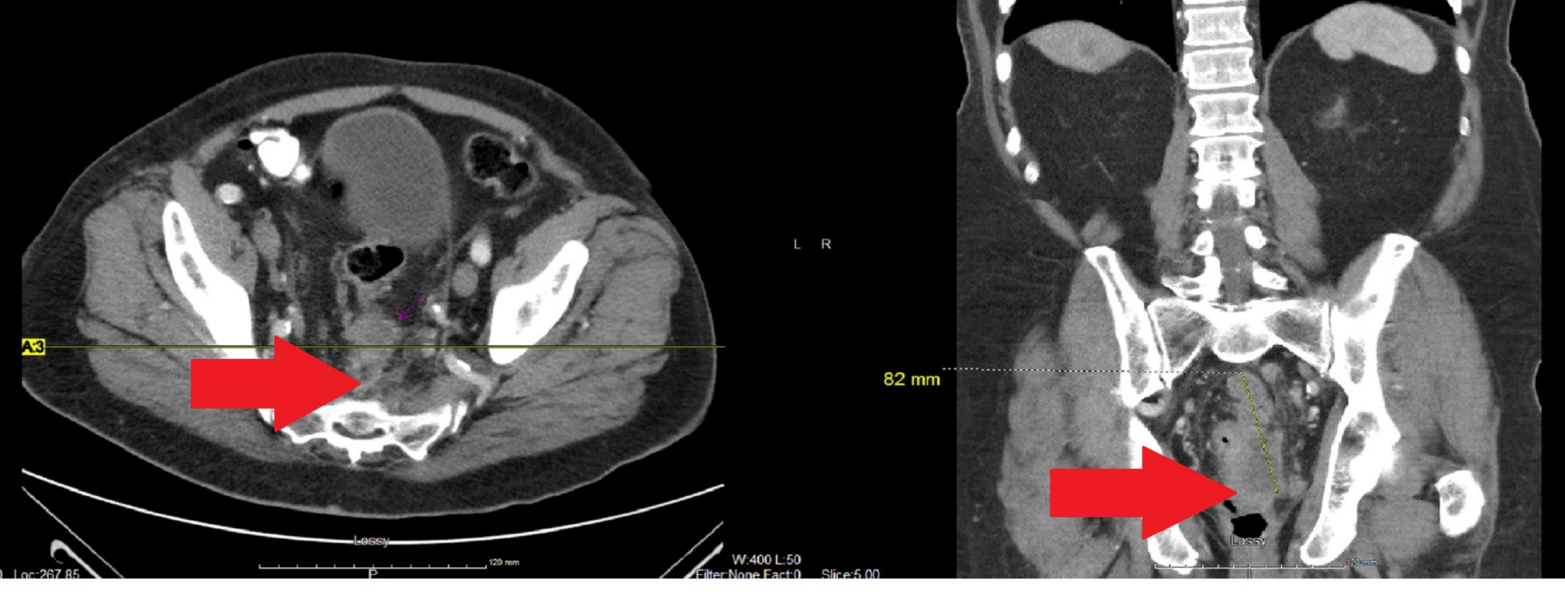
Computed tomography (CT) scan showing a rectal mass
